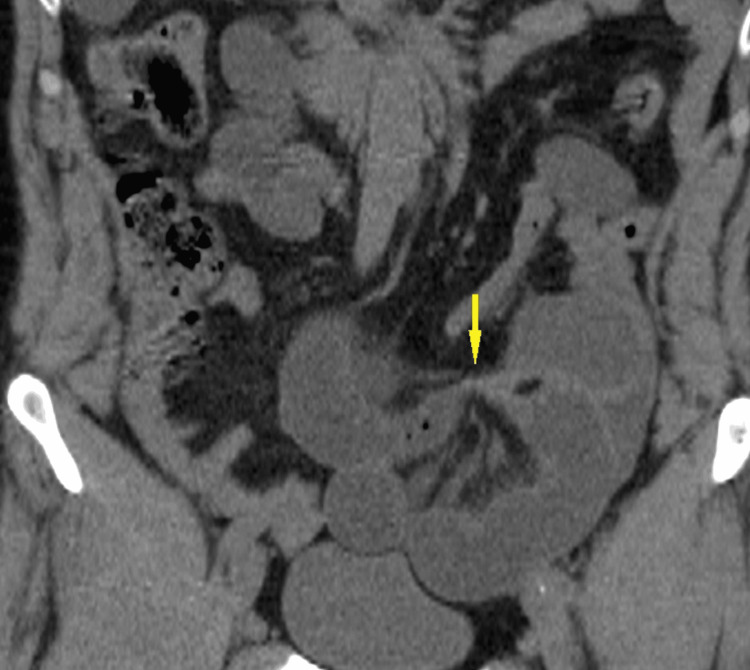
Coronal CT scan showing encapsulation and arrangements of distended small bowel loops withing abnormal sac in left iliac fossa giving -C- shape for the distended segment, suggesting an internal inter-sigmoid hernia. Yellow arrow: Zone of transition
